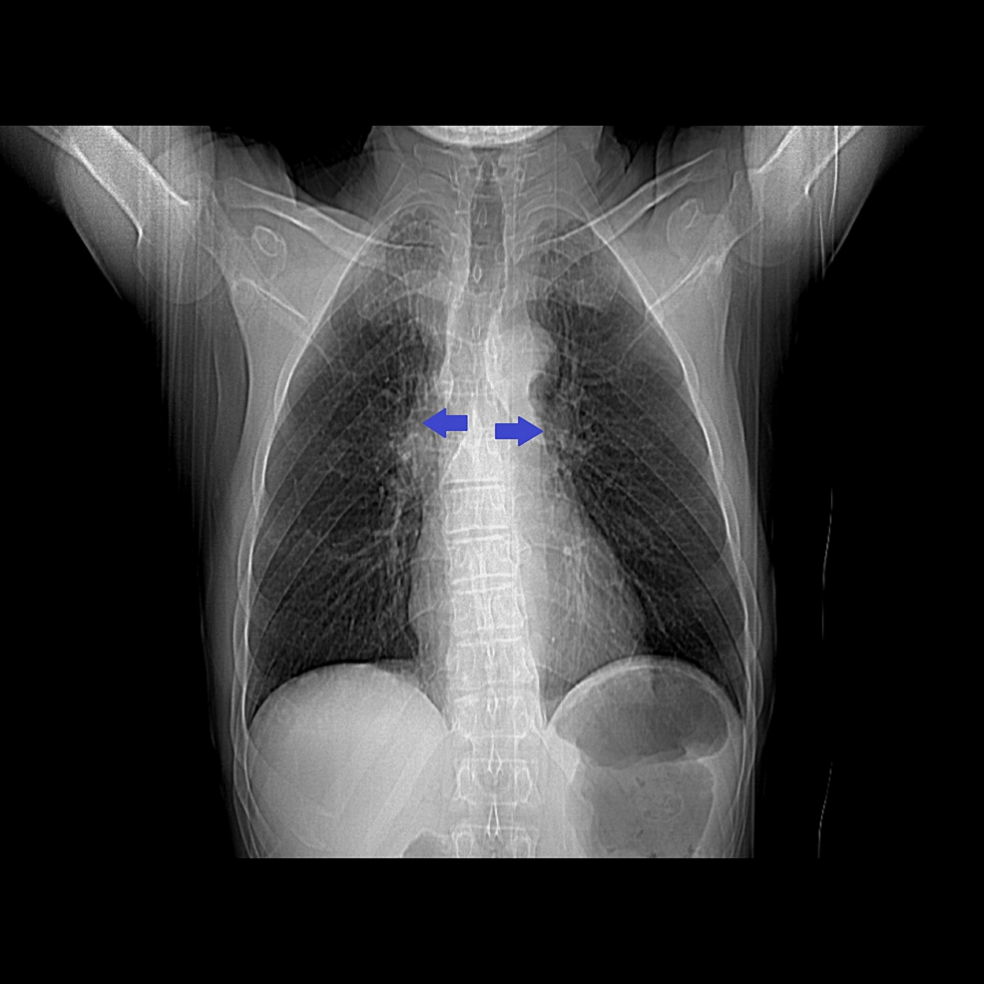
CT scout view of the chest showing an enlargement of the mediastinum (blue arrows).
